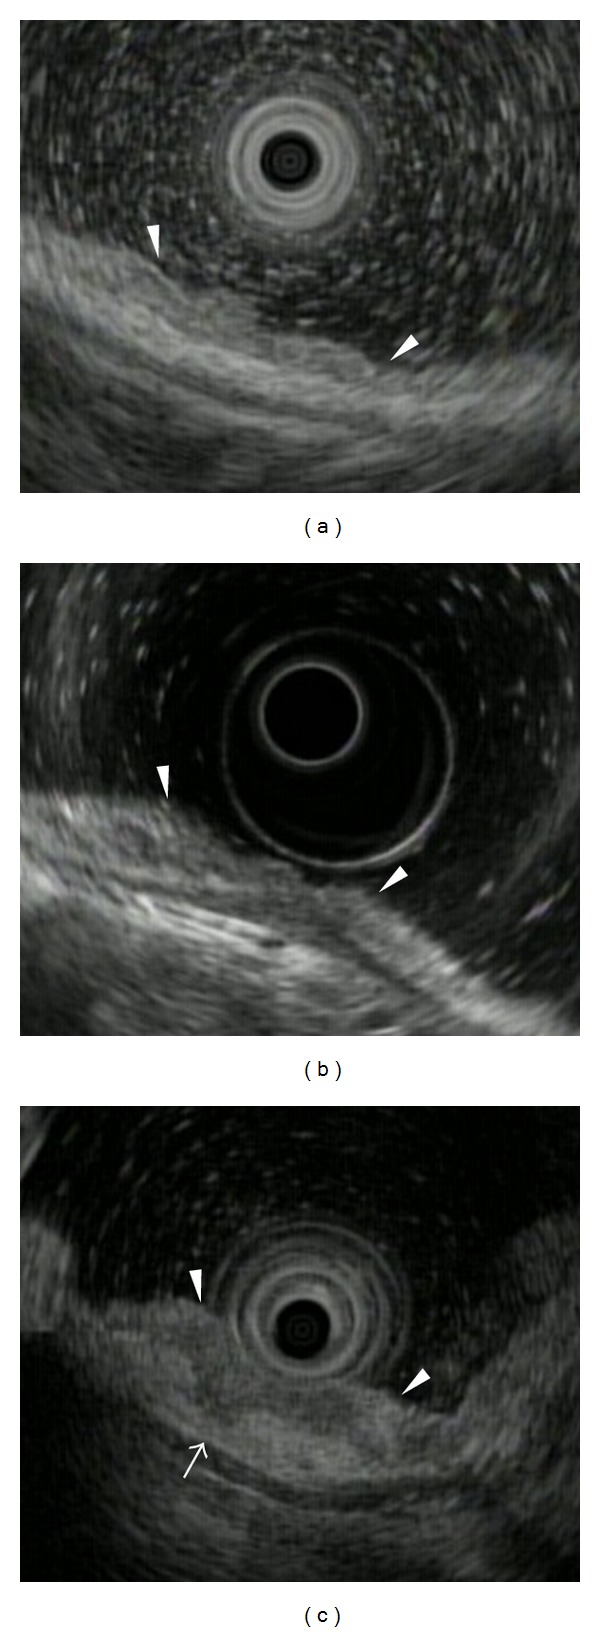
Endoscopic ultrasonography images of early gastric cancer. (a) EUS-M/SM1, type 0-IIc: there is no destruction in the first and second layers. The third layer looks normal. (b) EUS-M/SM1, type 0-IIc + III: the third layer shows smooth tapering and convergence. (c) EUS-SM2, type 0-IIa: a hypoechoic tumor shows the submucosal invasion. (white arrow).
